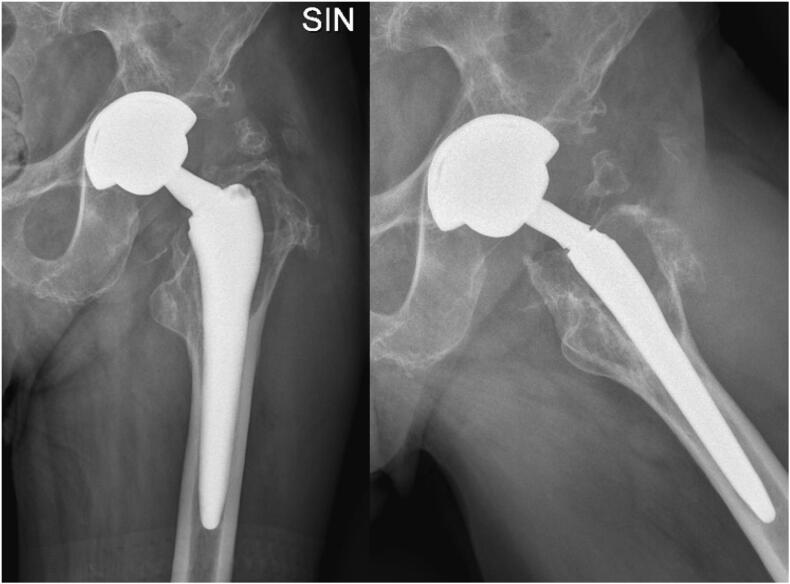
Preoperative X-rays (anteroposterior and lateral view) - bone resorption around the left femoral stem.
